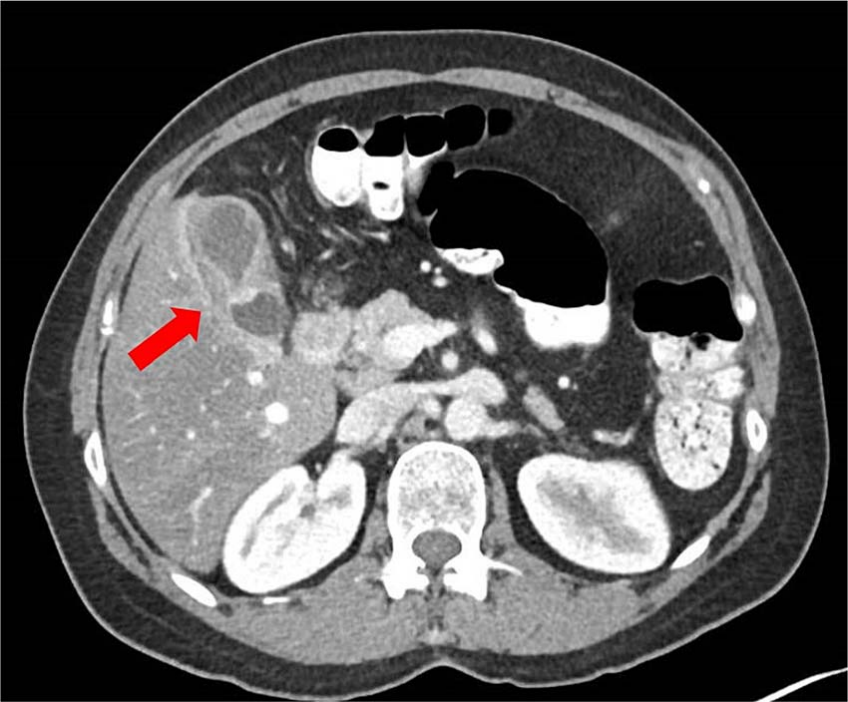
Abdominal CT scan showing an ‘hourglass’ appearance of the gallbladder (arrow).
